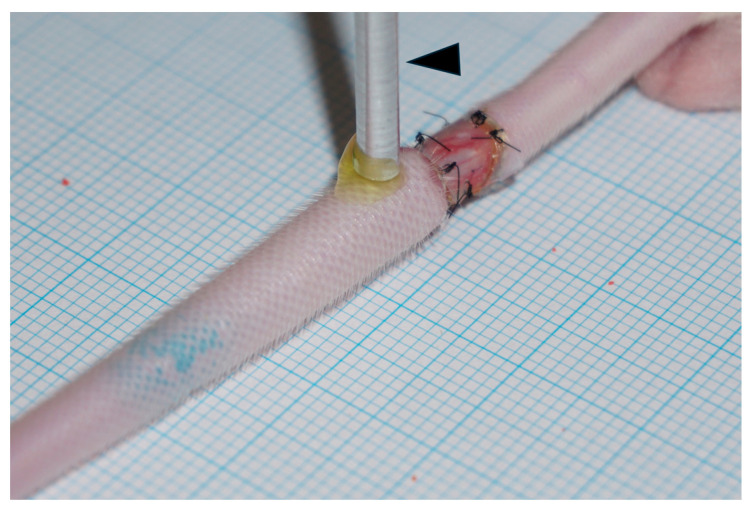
An image of ultrasound irradiation in progress. The black arrow indicates the ultrasound probe. FITC dextran or clobetasol propionate lotion was applied to the skin surface for the probe.
